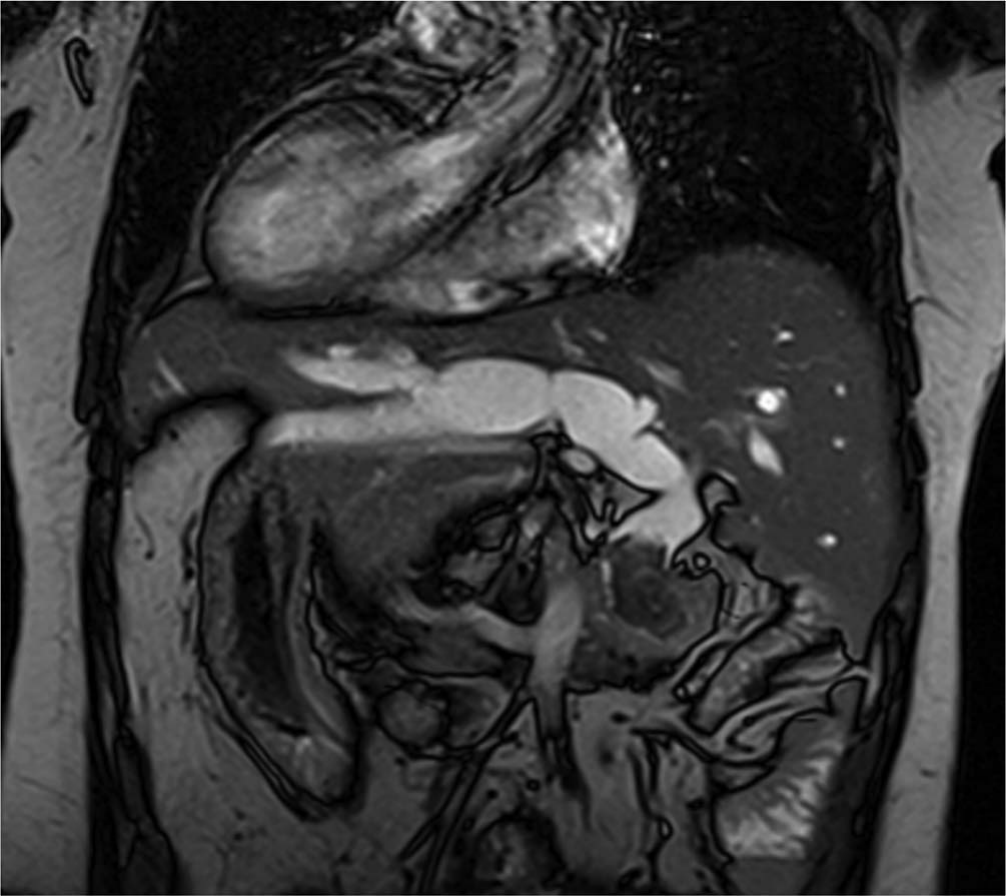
MRI shows a 26 mm stone in the distal common bile duct with bile duct dilation.
